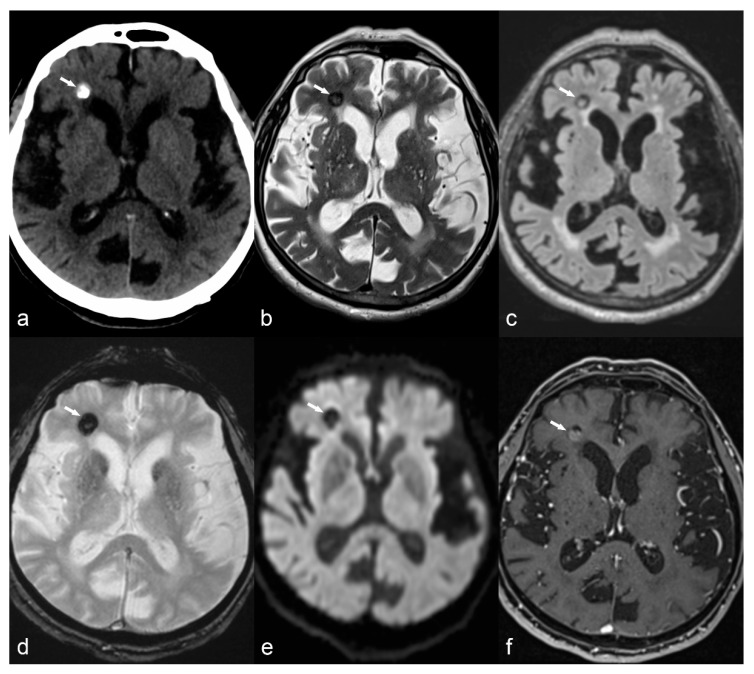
Right frontal cavernoma. Axial CT image (a) shows right frontal periventricular hyperdensity (arrow) abutting the frontal horn of the lateral ventricle. Axial T2 (b), FLAIR (c), GRE (d) and DWI (e) trace images (b = 1000) show peripheral hypointense rim with central hyperintensity (arrow). Axial T1 VIBE post-contrast image (f) shows mild enhancement (arrow) consistent with cavernoma.
